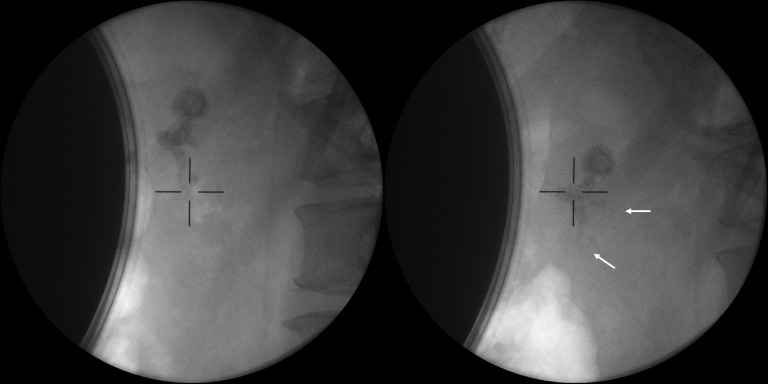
X-ray showed that stone fragments (arrows) were expelled after extracorporeal shock wave lithotripsy.
